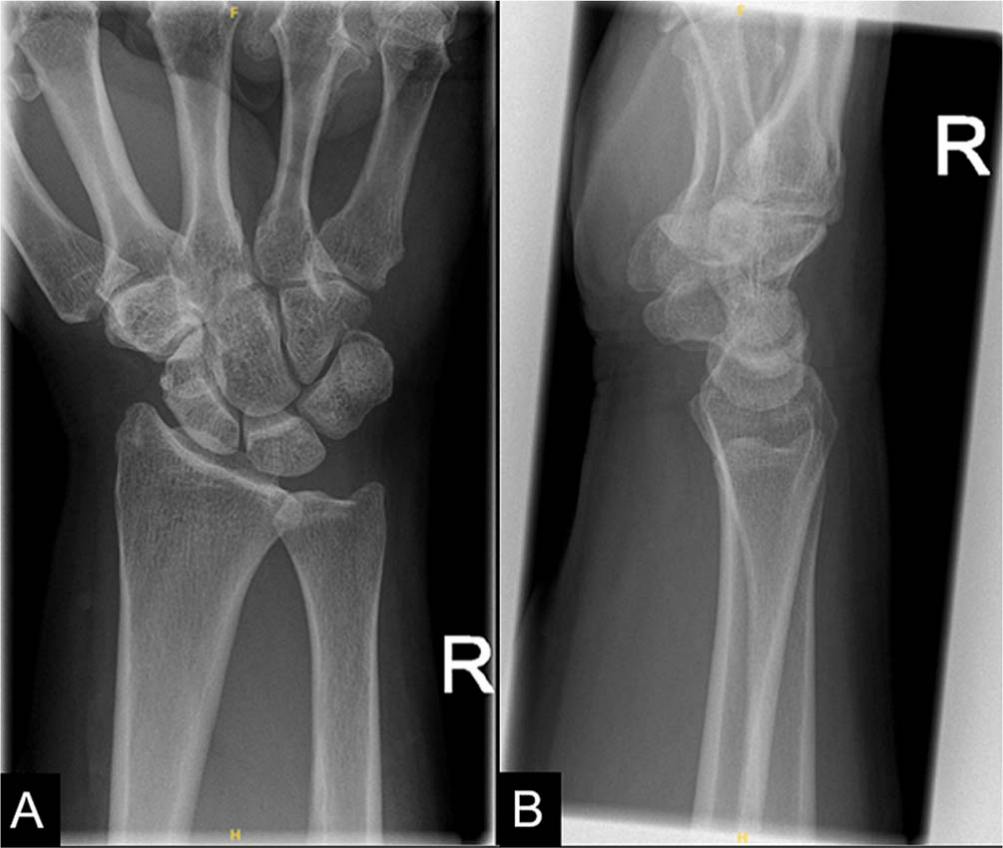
A repeat X-ray of the right wrist indicating that the previous calcific focus at the flexor carpi radialis tendon region had resolved.
